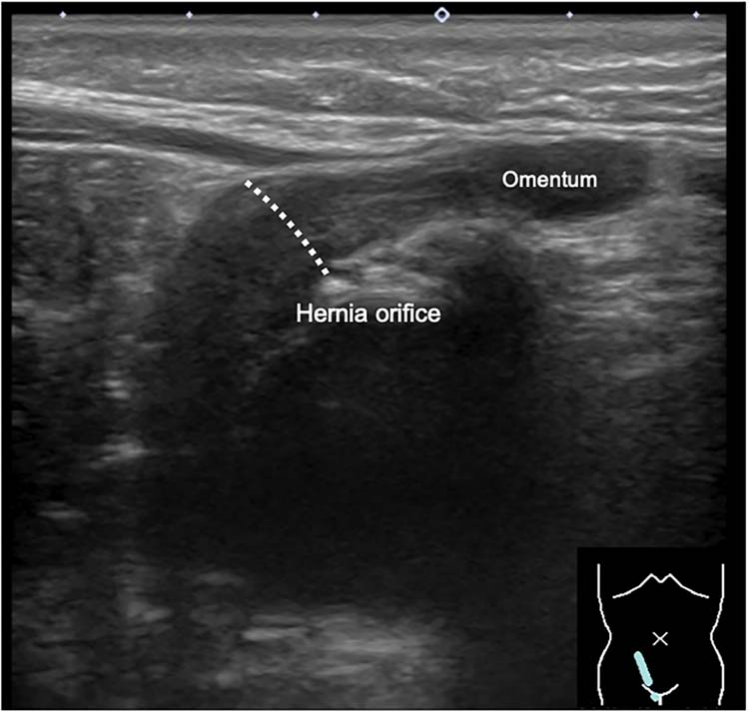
Inguinal ultrasound image captured at 11 months of age, showing herniation of the omentum but no apparent protrusion of the uterus.
